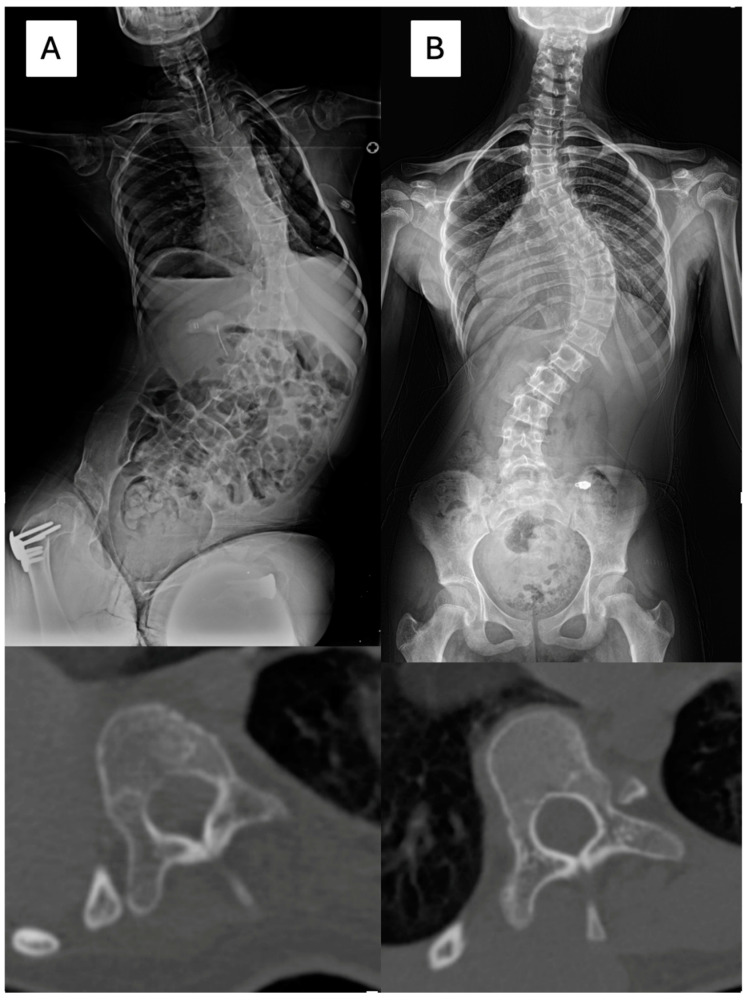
(A) A female aged 10 years and 7 months had neuromuscular scoliosis with spastic quadriplegic cerebral palsy. The Cobb angle between T6 and L4 was 79 degrees, and the apex was T10 (CT). The pedicle diameter and chord length were 3.2 and 24.9 mm at the concave side, and 4.8 and 29 mm at the convex side. (B) An 11 years, 6 months old female was diagnosed with adolescent idiopathic scoliosis with a Cobb angle of 74 degrees (T7-L1) and apex at T10 (CT). The pedicle diameter and chord length were 3.9 and 37.5 mm at the concave side, and 4.8 and 39 mm at the convex side.
